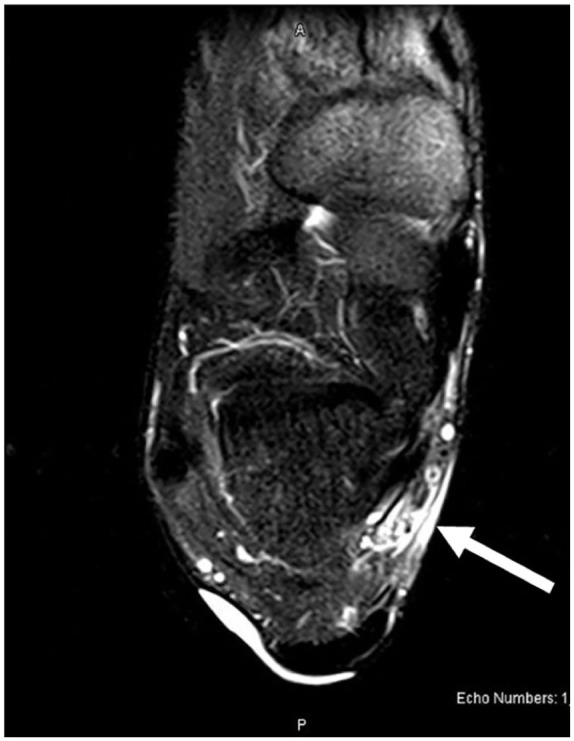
Magnetic resonance imaging: axial view.
